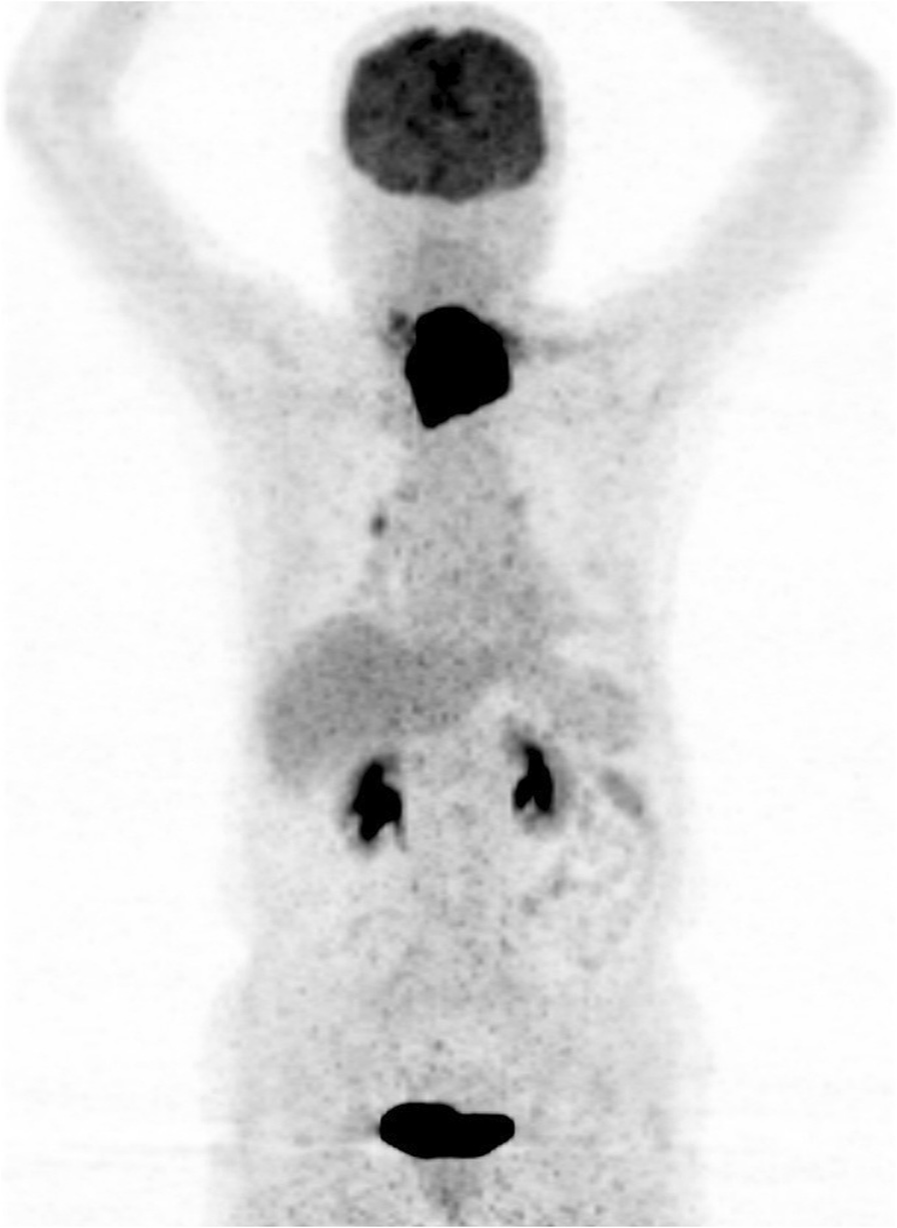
Whole body PET/CT scan evaluated the involvement. A hypermetabolic mass in the left supraclavicular fossa involved the left lobe of the thyroid. The large primary tumor compressed adjacent organs such as oropharynx, hypopharynx, larynx, trachea, and esophagus. No other abnormal FDG uptake lesions were found.
